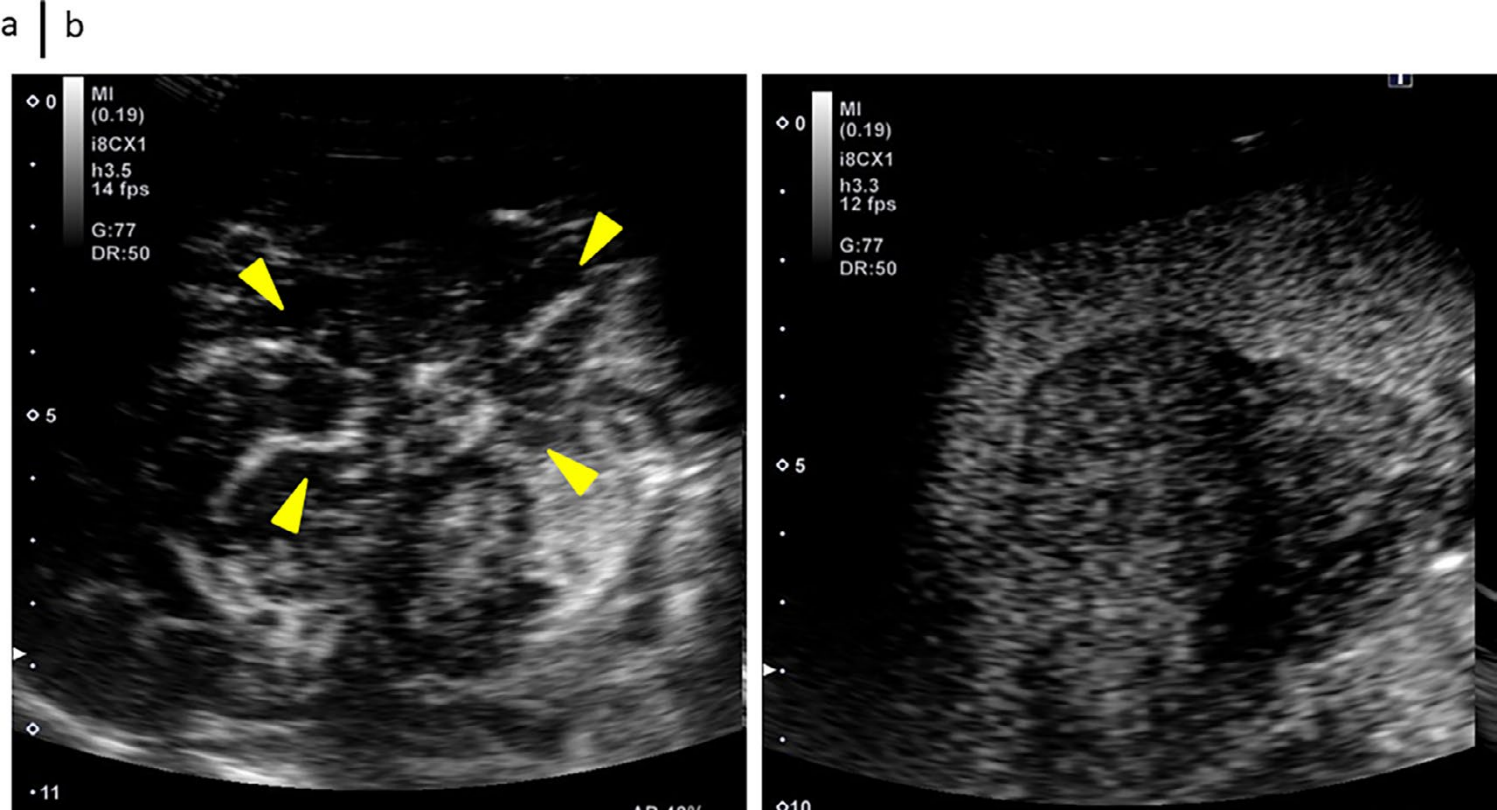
Contrast‐enhanced abdominal ultrasound examination after referral. (a) In the arterial‐dominated phase, it is extracted as a hypervascular mass, with some axle‐shaped blood vessel structures also observed (arrow). (b) In the Kupffer phase, it is extracted as a defect image.
